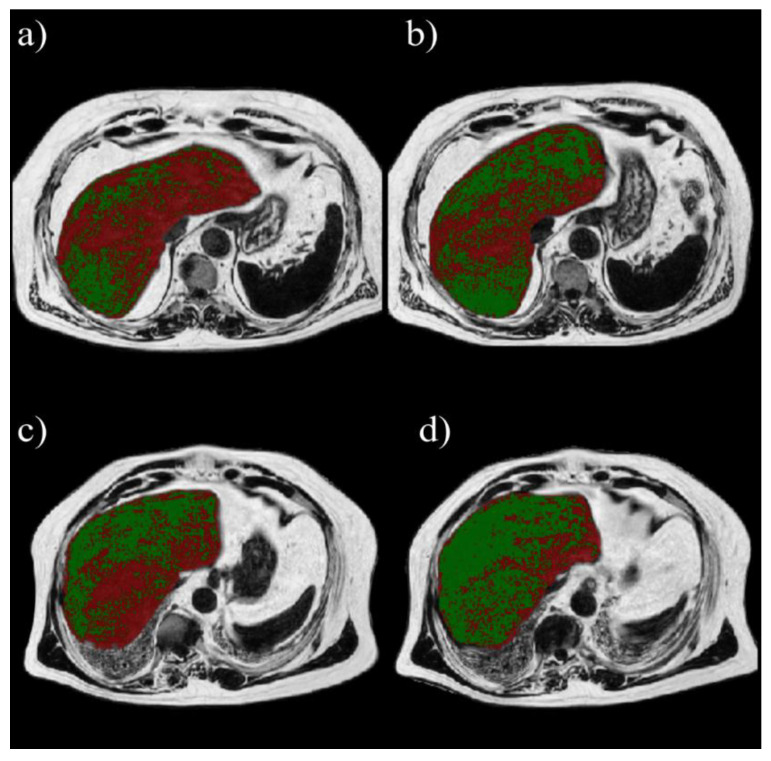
Examples of volumetric analysis. On the first row, a patient who underwent MUFA diet with percentage of liver volume with MRI-PDFF > 5.56% (red) decreasing post-treatment (b) with respect to pre-treatment (a) of 24.4%. On the second row, a patient who underwent multifactorial diet with percentage of liver volume with MRI-PDFF > 5.56% (red) decreasing post-treatment (d) with respect to pre-treatment (c) of 32.3%.
